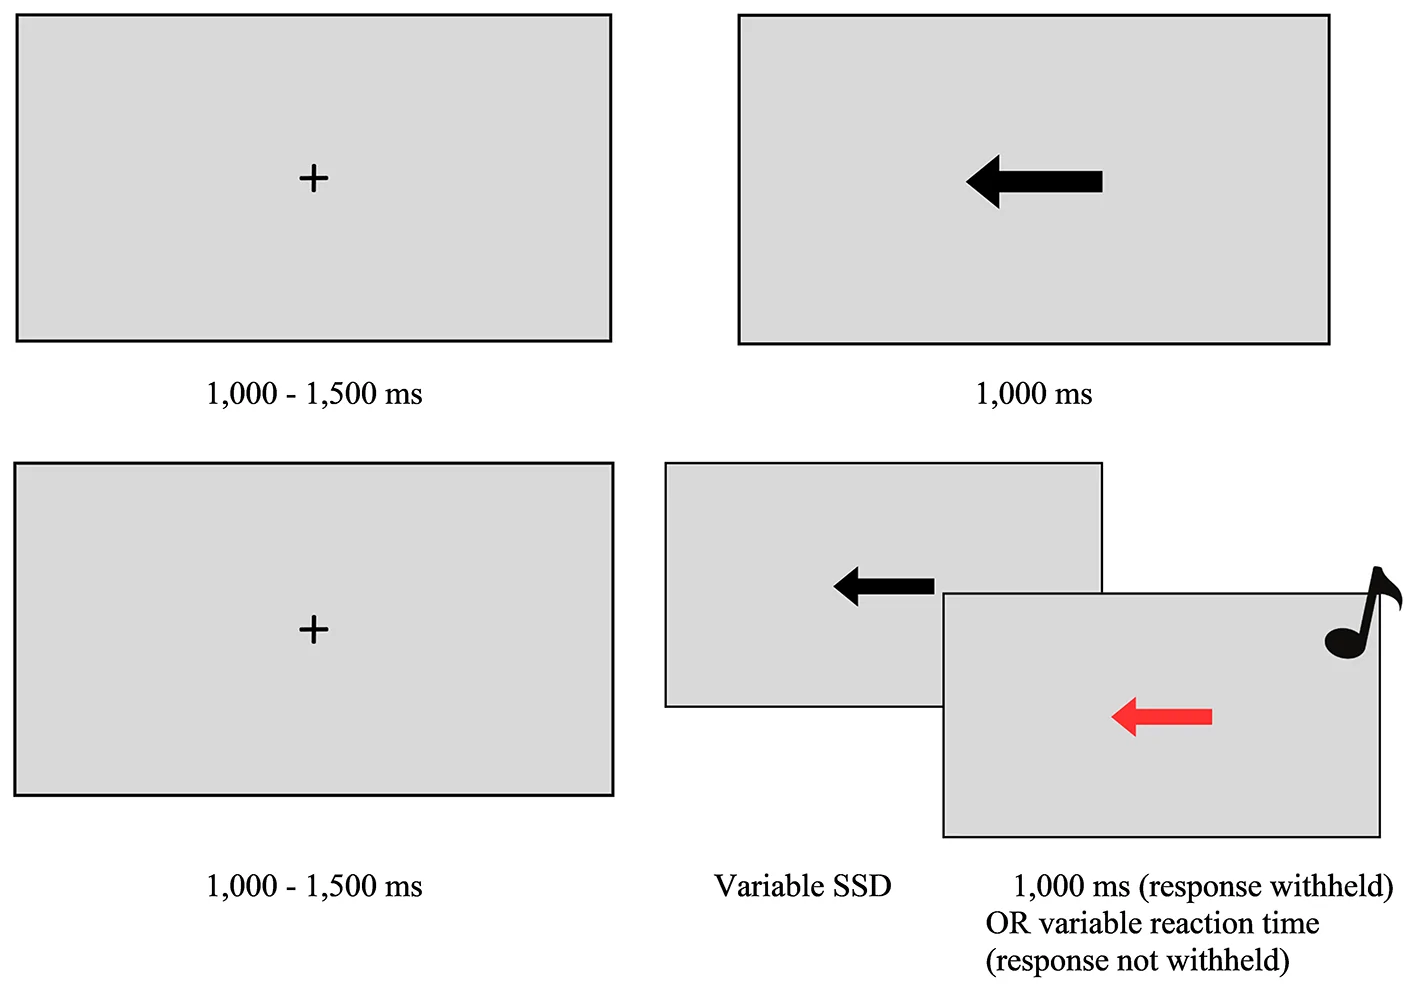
Procedure for the functional magnetic resonance imaging stop signal task. On “go” trials, a black arrow pointing left or right is presented for 1,000 ms, and participants indicate arrow direction using a button response. On “stop” trials, a “go” black arrow is initially presented and then replaced by a red arrow alongside an audio tone after a variable stop signal delay. Trials are preceded by a fixation cross of variable duration.
